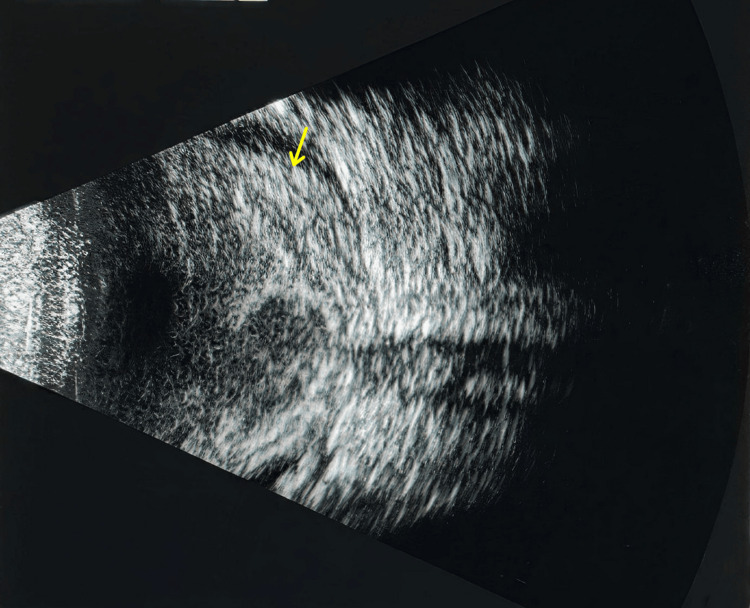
B-scan ultrasonography of the left eye at presentation B-scan ultrasonography of the affected left eye demonstrating markedly hyperechogenic vitreous with dense membranous echoes distributed throughout the vitreous cavity, consistent with severe vitritis (yellow arrow), with the retina remaining attached in all four quadrants.
